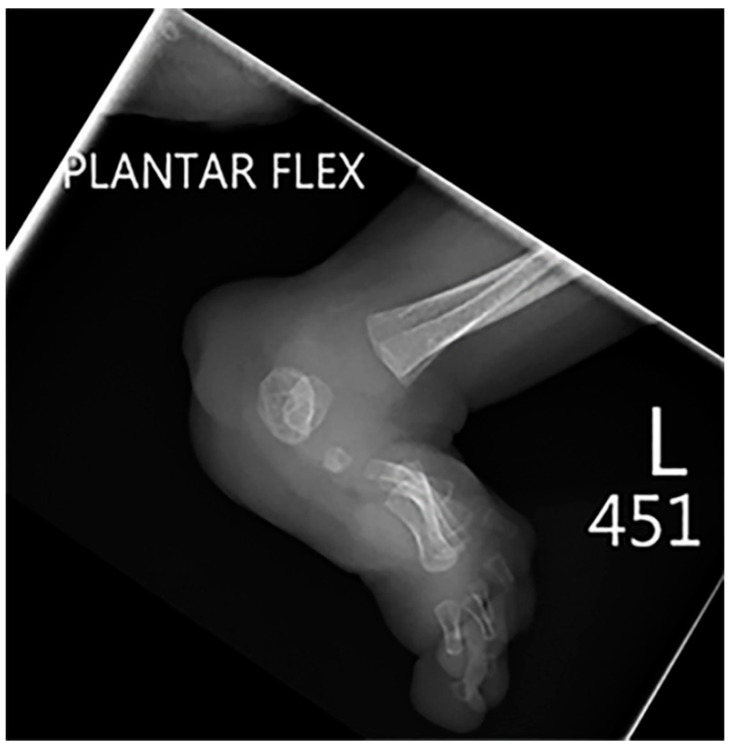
Lateral radiography of the same patient as shown in Figure 8 shows an extensive foot deformity.
